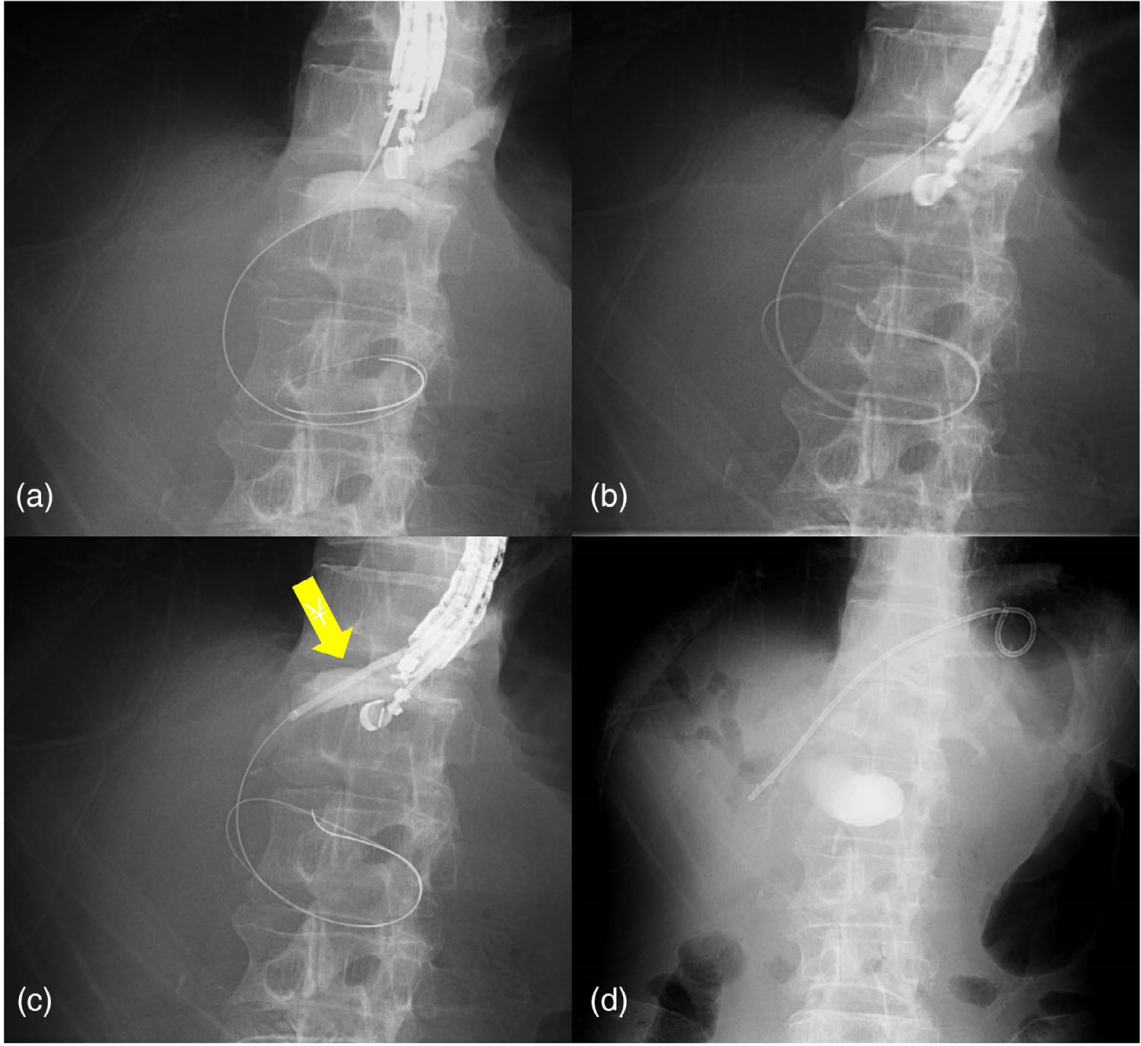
Tract dilation using a new balloon catheter in endoscopic ultrasound‐guided hepaticogastrostomy. (a) After puncturing B2, a 0.025‐inch guidewire was placed into the common bile duct. (b) A new long balloon catheter was inserted. (c) Tract dilation using the new balloon catheter. The inflated balloon is easily visible on the fluoroscopy screen (arrow). One‐step tract dilation of the gastric wall, liver parenchyma, and bile duct wall was achieved. (d) A 7‐Fr dedicated plastic stent for endoscopic untrasound‐guided hepaticogastrostomy was smoothly inserted.
